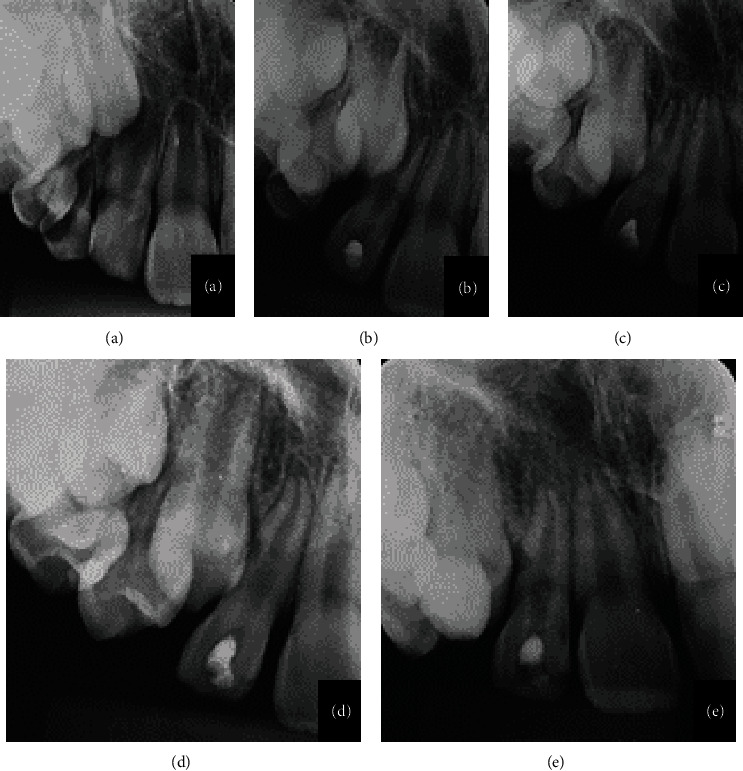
(a–e) 26-month radiographic follow-up: periapical radiography before endodontic access and root perforation (a); maxillary lateral incisor with evidence of coronal access (b); follow-up at 14 (c) and 20 (d) months; evidence of complete rhizogenesis (e).
